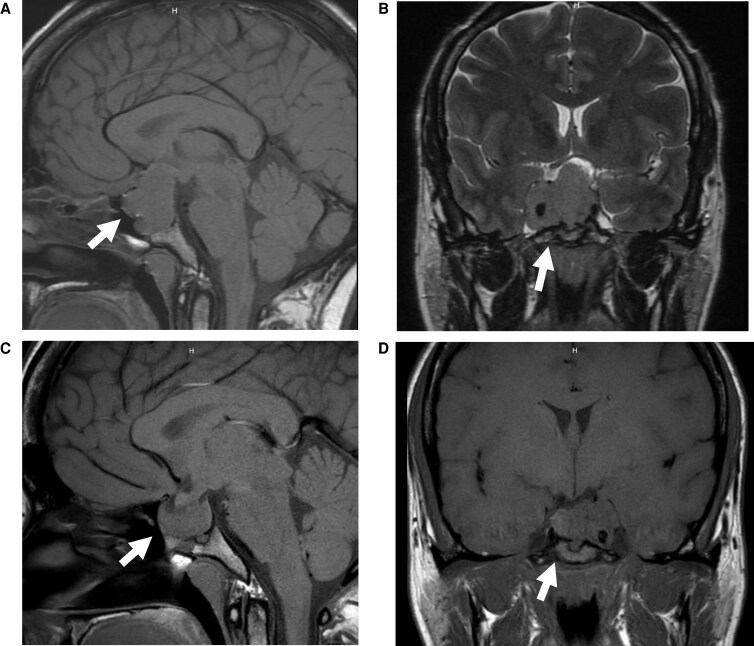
Pre- and postoperative MRI of the pituitary macroadenoma. Panel A, Preoperative sagittal MRI demonstrating a 3.5 × 3.2 × 2.8 cm sellar/suprasellar lesion with mass effect on the optic chiasm, invasion of the right cavernous sinus, and extension through the sellar floor into the sphenoid sinus. Panel B, Preoperative coronal MRI demonstrating tumor invasion into the right cavernous sinus. Panel C, Residual adenoma within the sella and extending posteriorly toward the prepontine cistern. Panel D, Postoperative coronal MRI showing decreased bulk of residual pituitary tumor in the right cavernous sinus.
